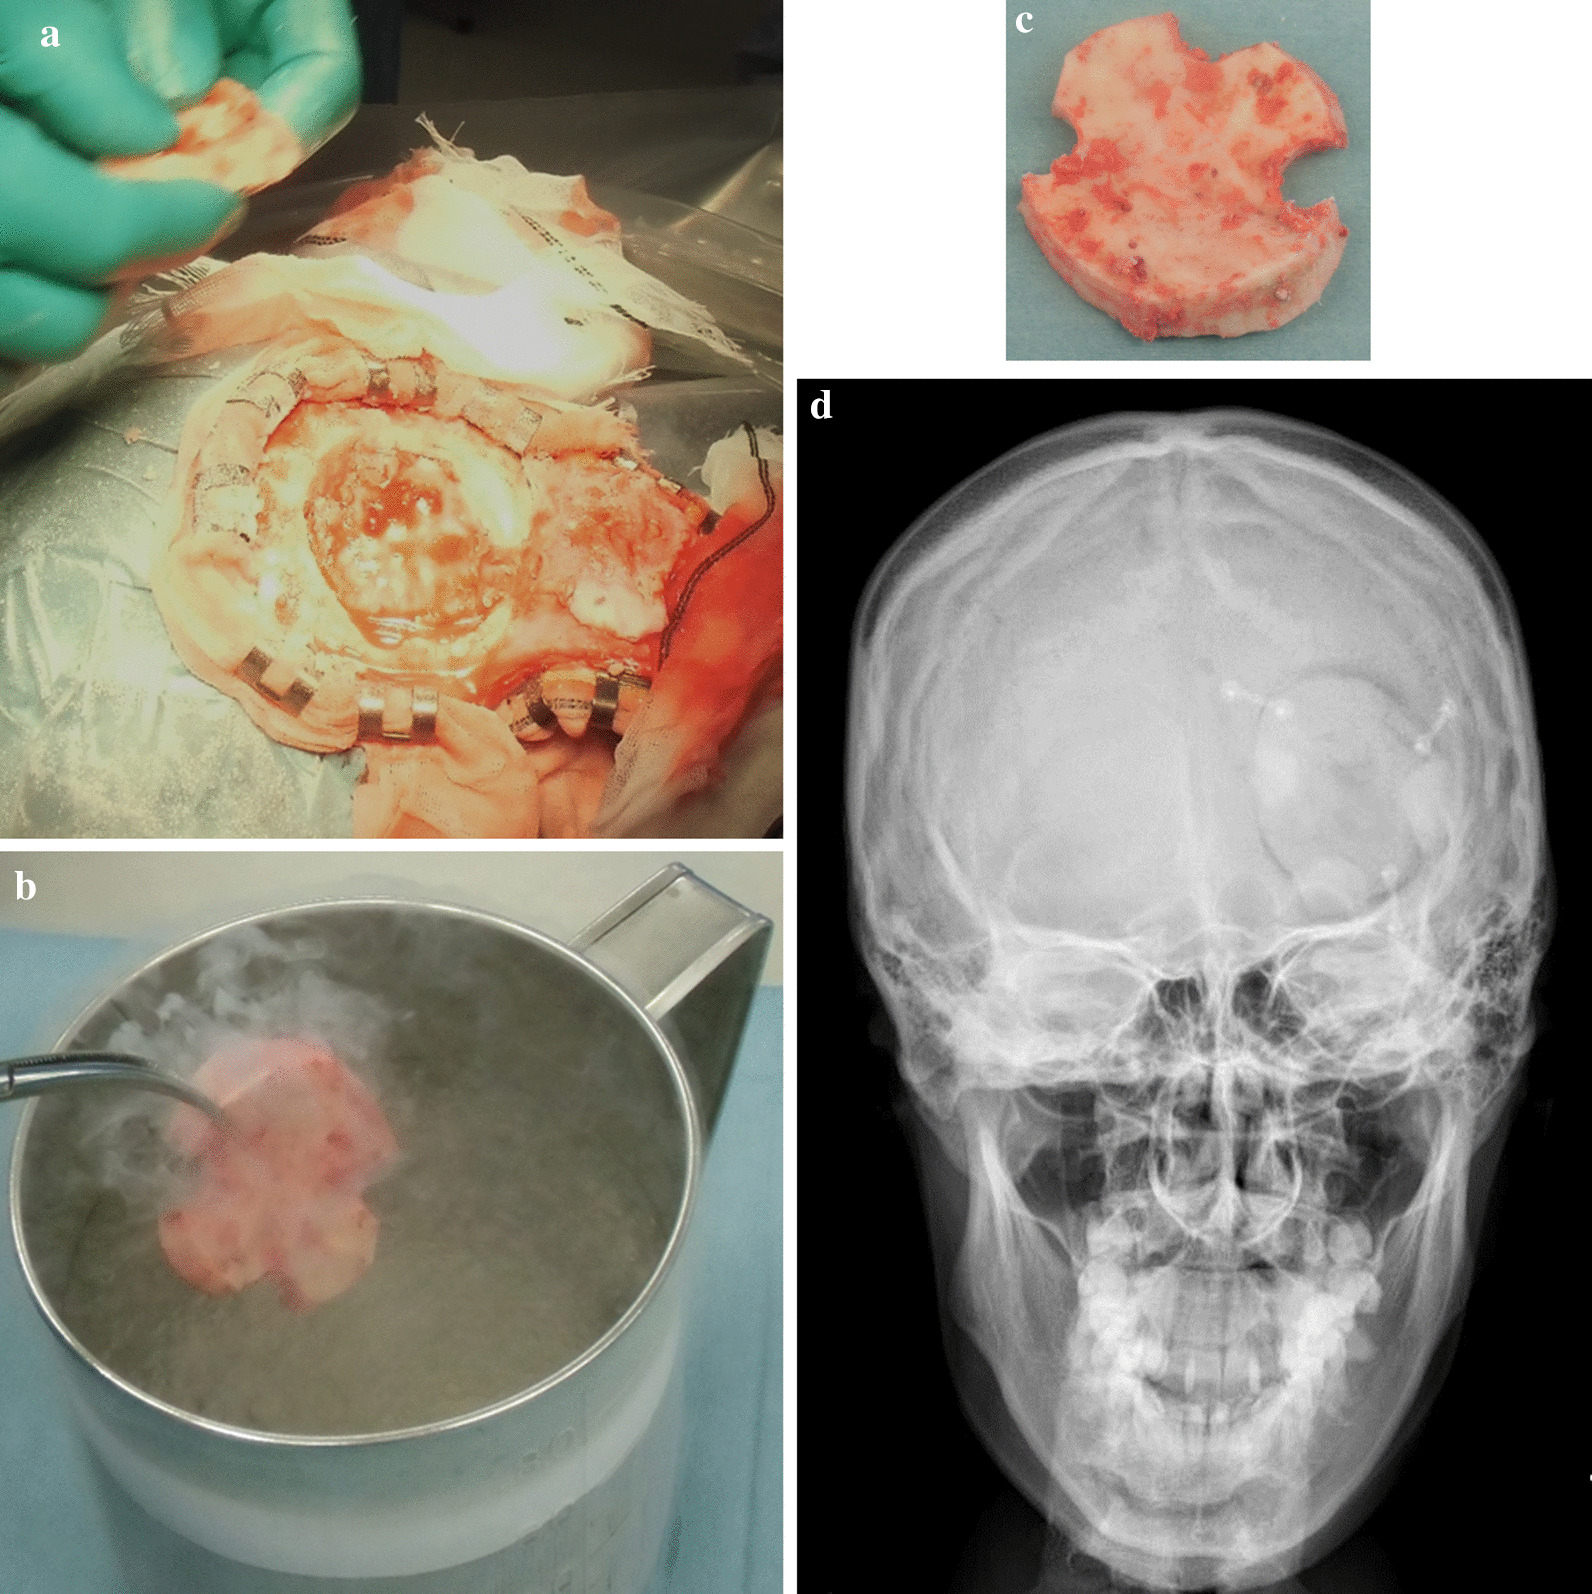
Surgical treatment for a skull lesion and the postoperative roentgenogram. a Skull lesion was excised with wide margin. b, c The excised bone was treated with liquid nitrogen for twenty minutes (b), and dissolved at room temperature for 15 min, and then was washed with 0.3% iodine saline and distilled water (c). d The frozen bone was returned to the original position and plate fixation was performed. Postoperative roentgenogram was shown
